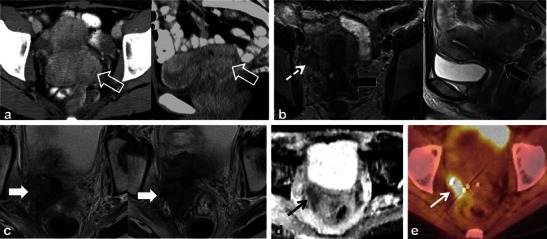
A 34-year-old woman with locally advanced cervical squamous cell carcinoma. Baseline pre-treatment axial and sagittal CECT (a) images demonstrate a large cervical tumour (*open white arrows*). Post-RT follow-up pelvic MRI performed 8 months later (b) depicts imaging findings consistent with complete response with reconstitution of the normal cervical zonal anatomy and homogeneous low T2 signal (*wide black arrows*) on axial and sagittal T2 TSE sequences. Note the small size and homogeneous low T2 signal without follicular activity in the right ovary (*dashed white arrow*) an expected post-RT finding. Follow-up pelvic MRI was performed 7 months later, axial T2 TSE images (c) reveal a heterogeneous ill-defined mass in the right parametrium that extends to the right pelvic side wall with areas of both intermediate and low T2 signal intensity which is suspicious for locally recurrent tumour (*solid white arrows*). In the early post RT setting immature fibrosis could have a similar appearance. DWI performed at same imaging session demonstrates restricted diffusion within this mass as low signal intensity on ADC map (*black arrow* in d). Subsequently whole-body PET-CT was performed (e) for the assessment of systemic disease, which depicts avid FDG uptake in the mass on fused axial FDG PET-CT image (*white arrow* in e). Biopsy was performed which confirmed recurrent tumour in this case involving both the central and lateral pelvic compartments
